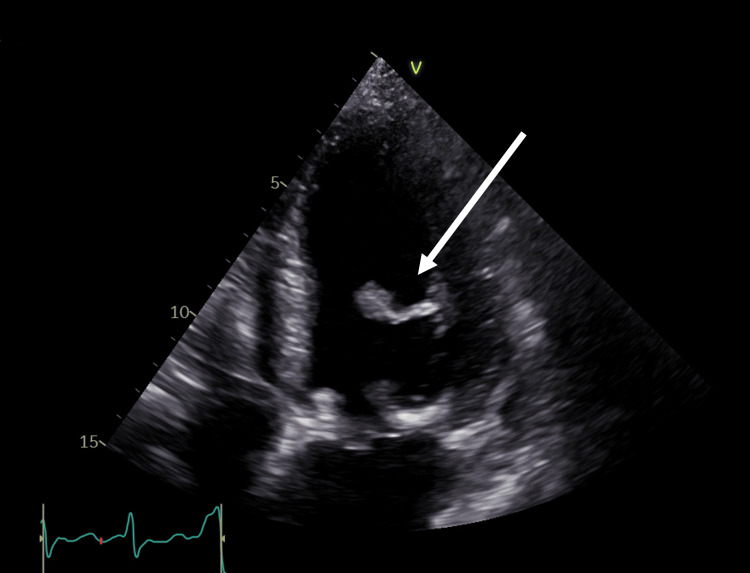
Transthoracic echocardiography showing papillary muscle rupture Apical four-chamber view reveals a ruptured papillary muscle within the left ventricle, contributing to severe postoperative mitral valve dysfunction and left ventricular systolic impairment
